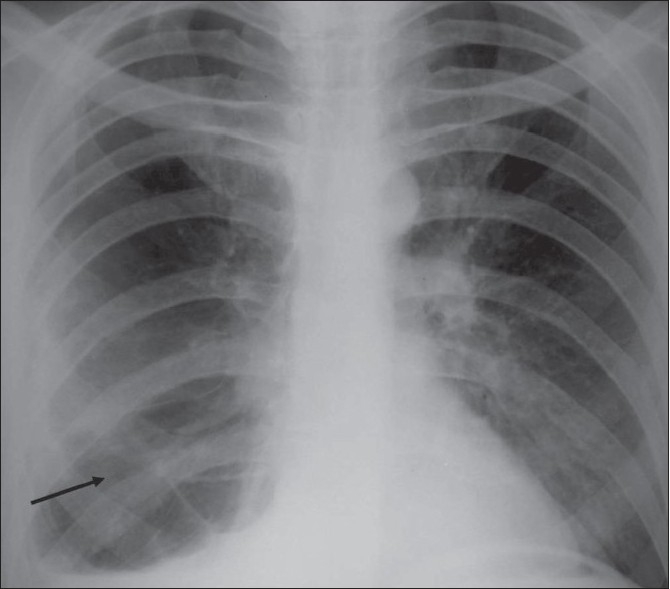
Chest radiograph done one month prior to presentation shows multiple bullae in the mid and lower zones (arrow) of the right lung with a blunted right costophrenic angle due to pleural thickening
